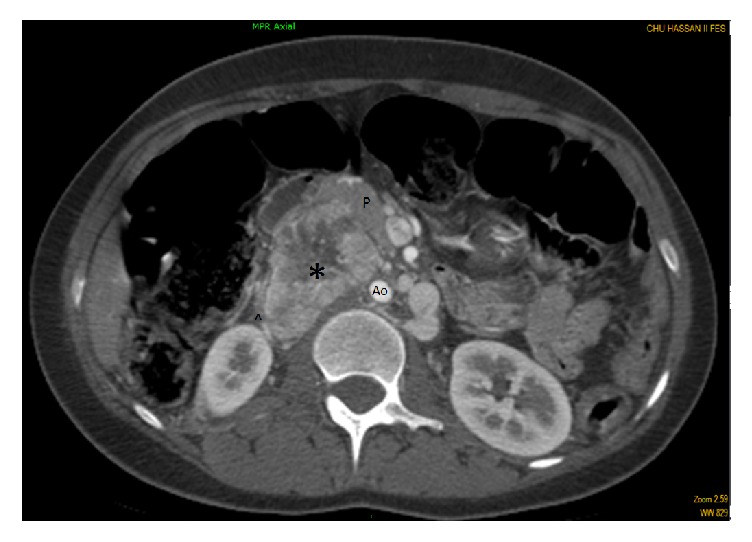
A contrast abdominal CT scan showing a 7.0 x 5.0 cm heterogeneous mass (black asterisk) located between the abdominal aorta (Ao) and the inferior vena cava, pushing the head of the pancreas (p).
